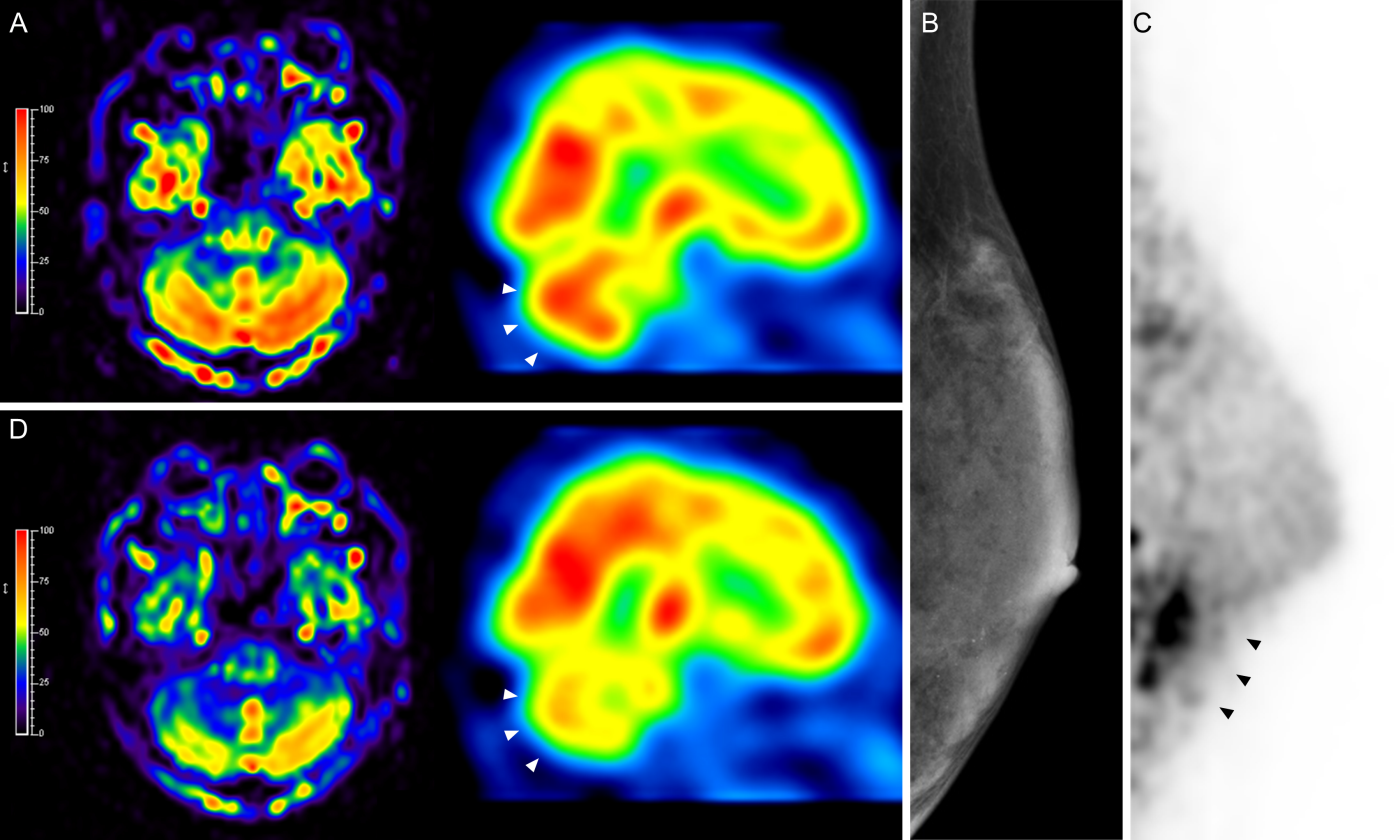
(A) Brain single photon emission computed tomography (SPECT) presented increased cerebellar perfusion (left: coronal; right: sagittal). (B) Mammography did not detect abnormalities. (C) Dedicated breast positron emission tomography presented an abnormal ^18^F-fluorodeoxyglucose uptake in the left breast. (D) Brain SPECT showed a decreased intensity of cerebellar hypermetabolism one week after surgery.
